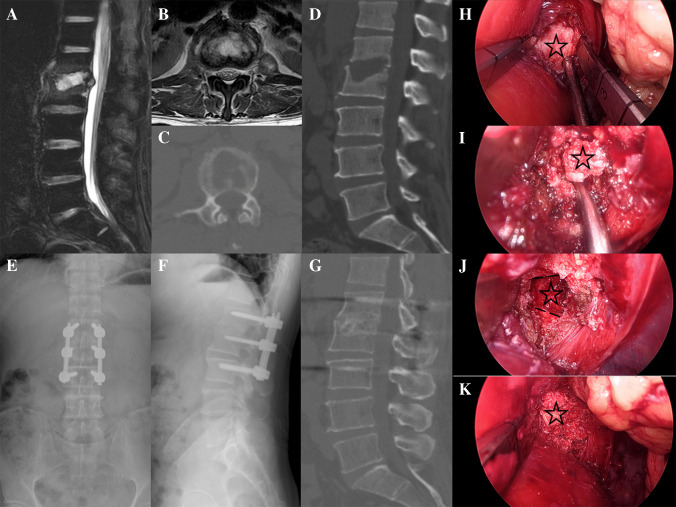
Case 3 A/B: Preoperative MRI demonstrates a lesion at the L1/2 level with an associated abscess causing spinal cord compression slightly. C/D: Preoperative CT reveals severe destruction of the L1/2 vertebral bodies. E/F:X-ray at 1 month postoperatively. G:CT at 12 months postoperatively confirmed complete fusion at L1/2. H: Exposure of the lesion, with the lesion marked by a black pentagram. I: Clearance of the lesion. J: Preparation of the bone graft bed, with the bone surface indicated by a black dashed line. K: Implantation of allogeneic bone
